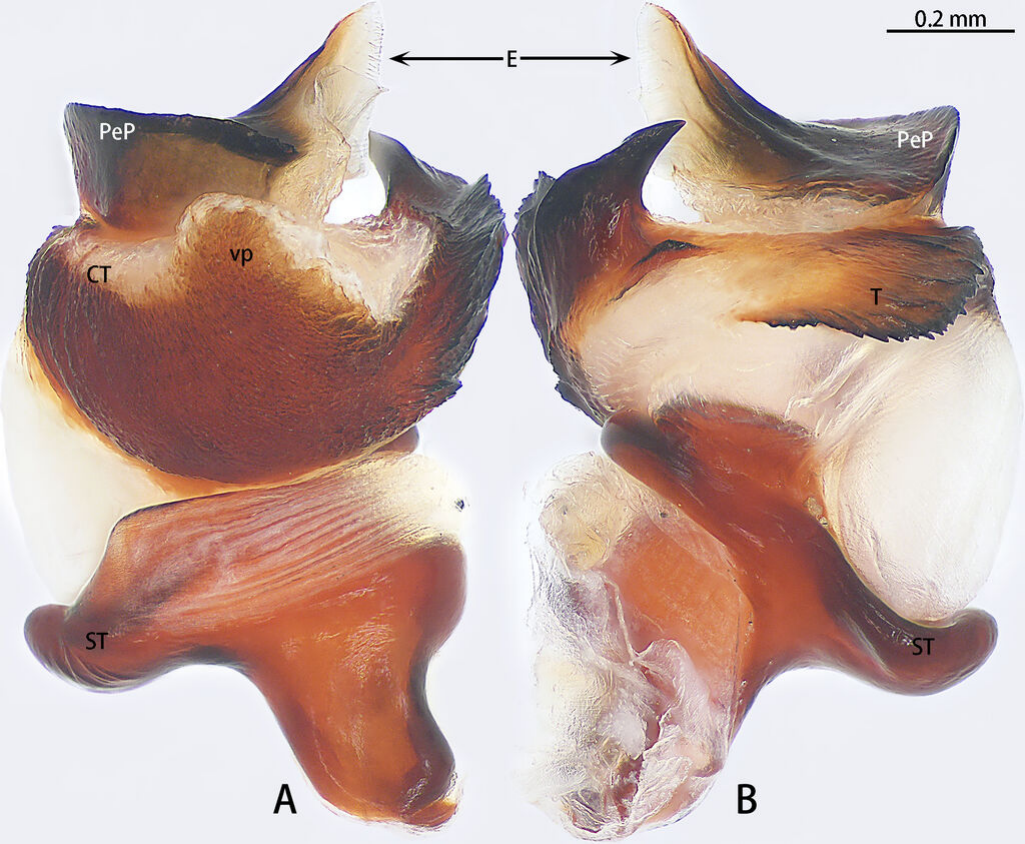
Dissected bulb of *Liphistiusliz* sp. nov., holotype male. A prodorsal view; B ventral view. Abbreviations: CT, contrategulum; E, embolus; PeP, paraembolic plate; ST subtegulum; T, tegulum, vp, ventral process of contrategulum.
